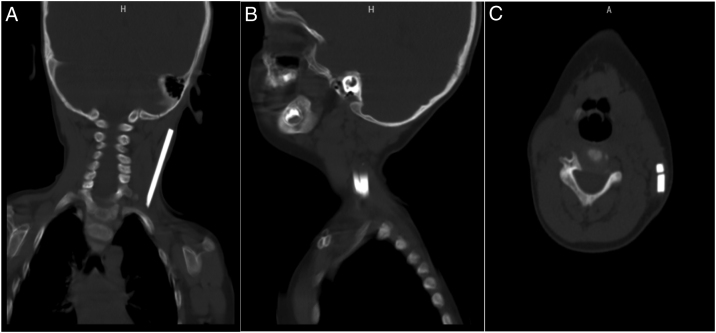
(A) Preoperative foreign body in neck CT images coronal section; (B) Preoperative foreign body in neck CT images sagittal section; (C) Preoperative foreign body in neck CT images axial section.
